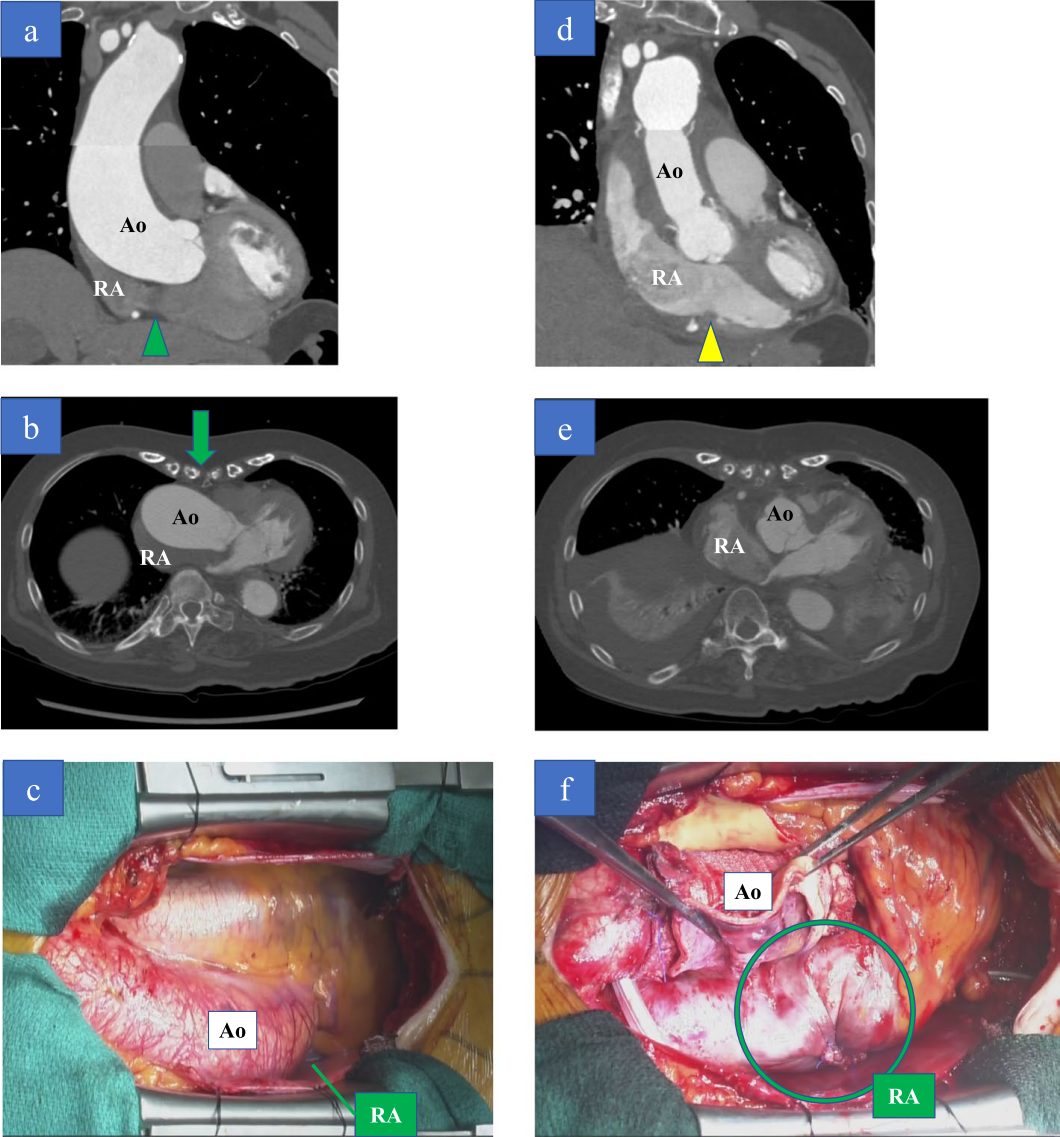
a and b Preoperative contrast-enhanced computed tomography shows deformity of the right atrium and narrowing of the tricuspid valve tract compressed by ascending aortic dilation and elongation and pectus excavatum (green arrowhead). d and e Postoperative contrast-enhanced computed tomography shows that the right atrium and tricuspid valve tract are not compressed by the ascending aorta (yellow arrowhead). c and f Before the replacement of the ascending aorta, the right atrium was compressed and hidden by a dilated and elongated ascending aorta (c). After the replacement of the ascending aorta, the RA is no longer compressed (f). Ao, Aorta; RA, right atrium
